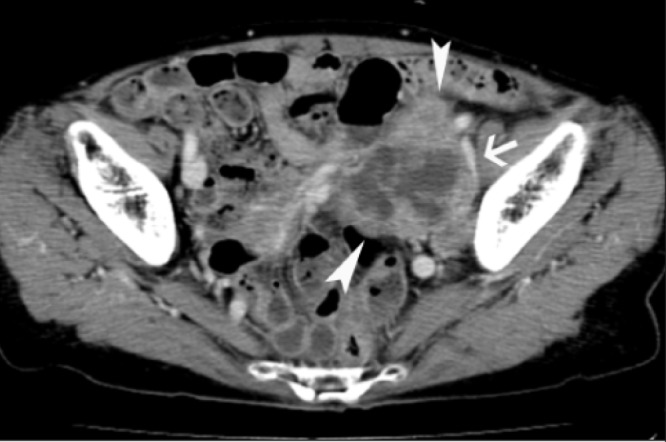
Post-contrast computed tomography of the abdomen at presentation showing a lobulated mass (arrowheads) with cystic components in the left pelvic wall. The mass encases the left iliac vein (arrow).
